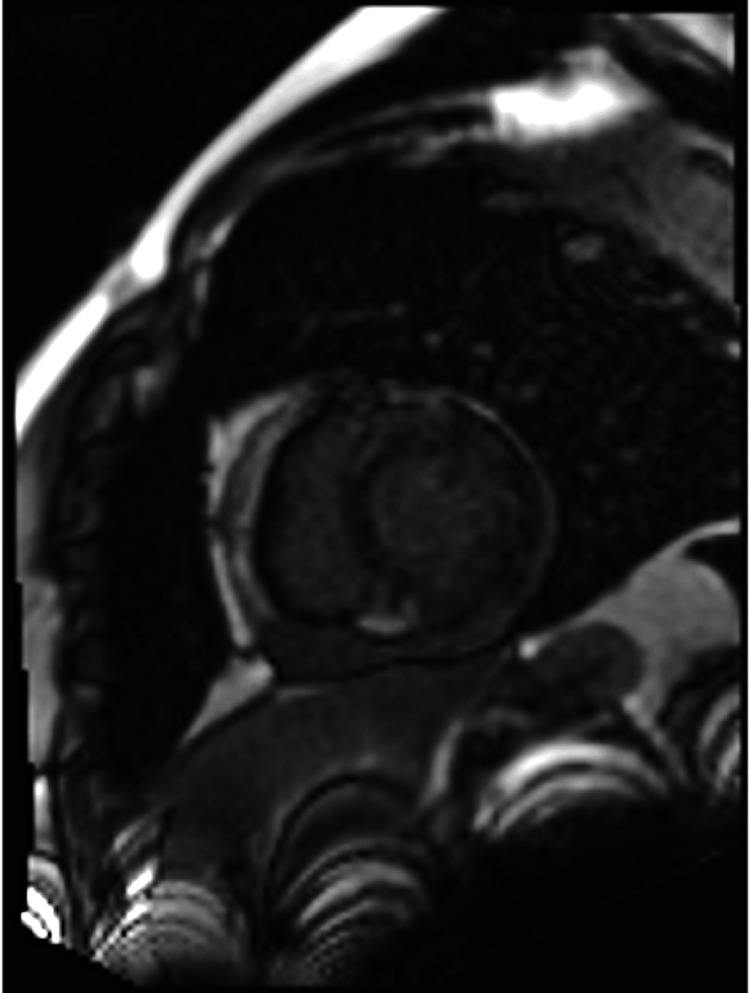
Initial MRI showing late gadolinium enhancement
